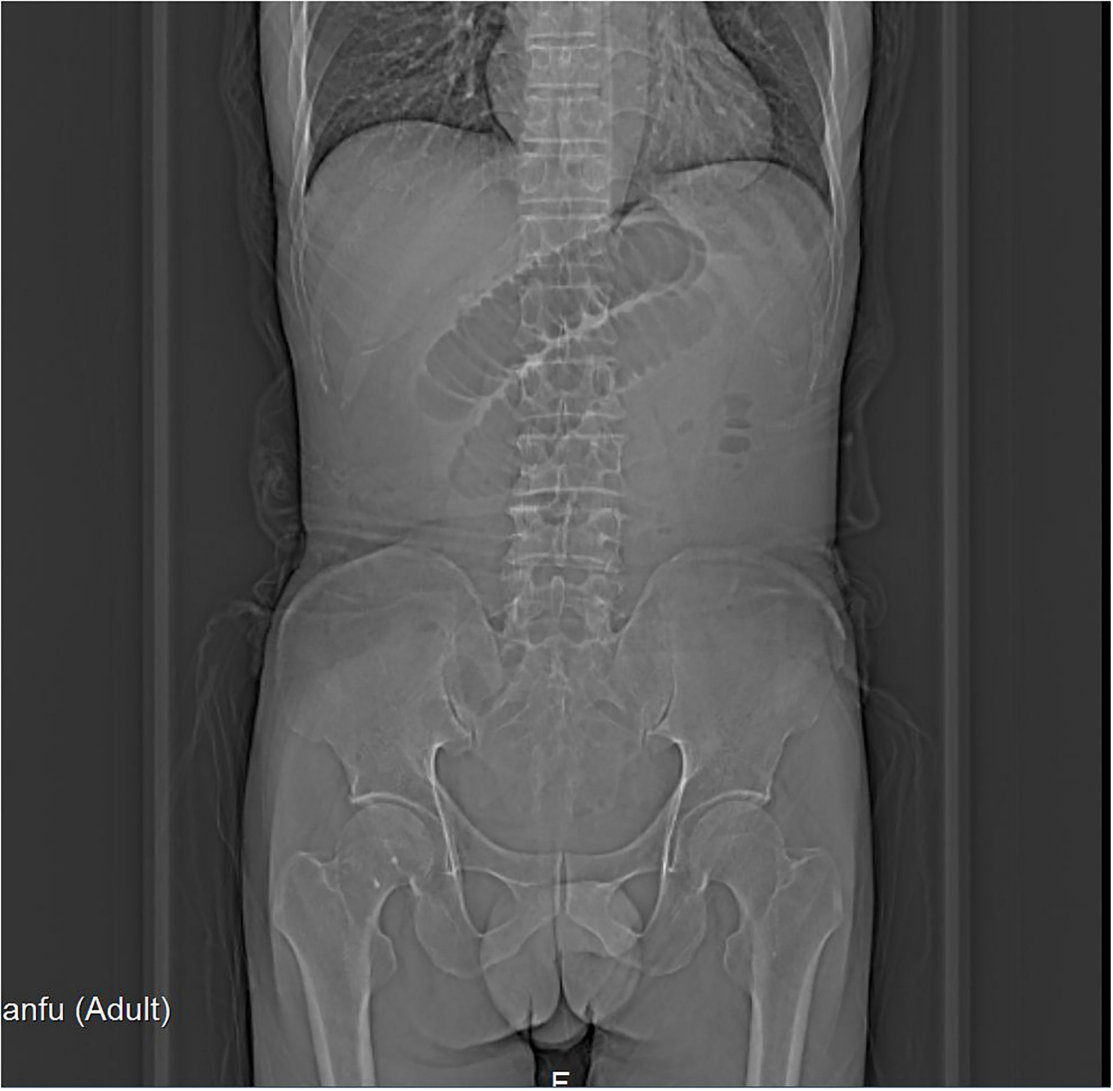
Abdominal X-ray showed intestinal obstruction.
